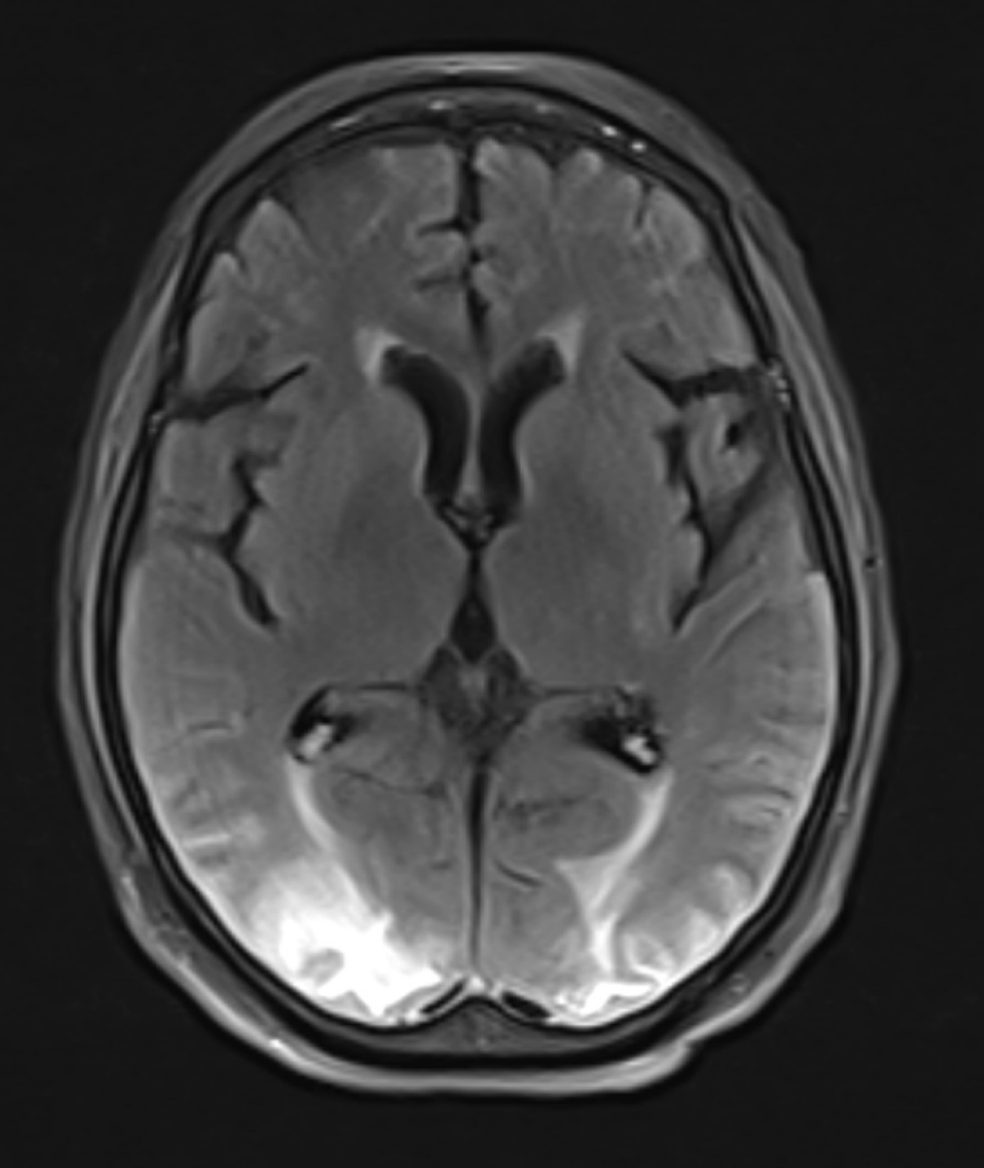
T2/FLAIR hyperintensities of bilateral occipital lobes. FLAIR: fluid-attenuated inversion recovery
